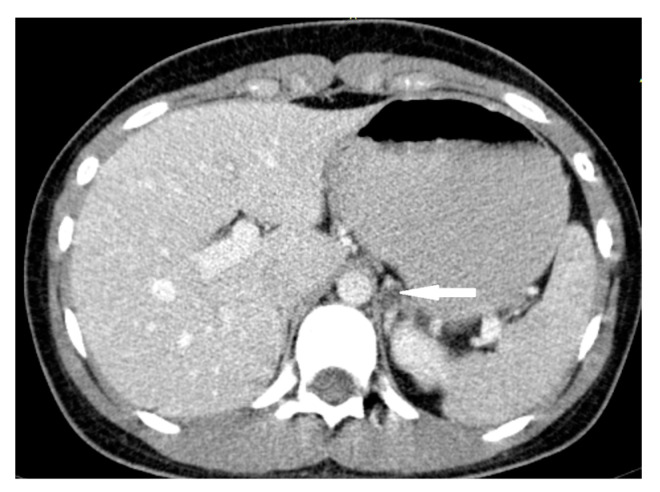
Adrenal lymphangioma. Abdomen CT, transverse cross-section.
